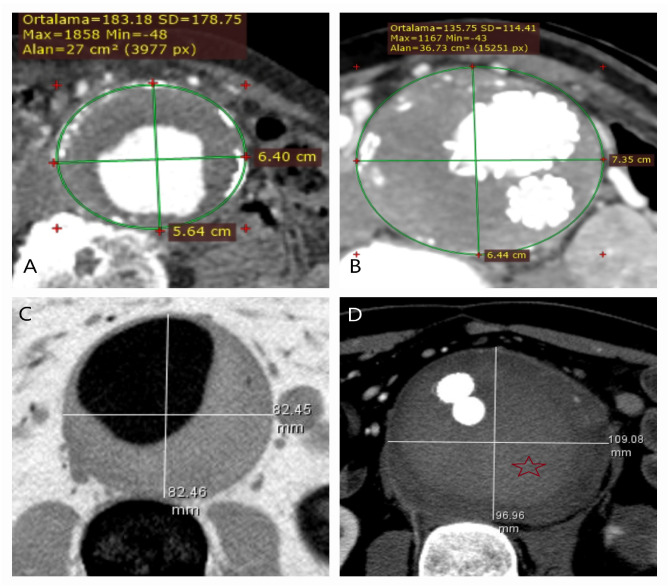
CT images demonstrating aneurysm sac enlargement after EVAR. (A,B) A 73-year-old male developed limb graft migration due to progressive iliac artery dilation, leading to a Type 1b endoleak and a 1 cm sac expansion two years post-EVAR. (C,D) A 60-year-old male with coronary artery disease and COPD experienced persistent abdominal pain one year after EVAR, revealing a 2 cm sac enlargement. A hypodense area within the sac, without extraluminal contrast, indicated a Type 5 endoleak (red star). In both cases, continued smoking and poor adherence to antihypertensive therapy contributed to disease progression.
